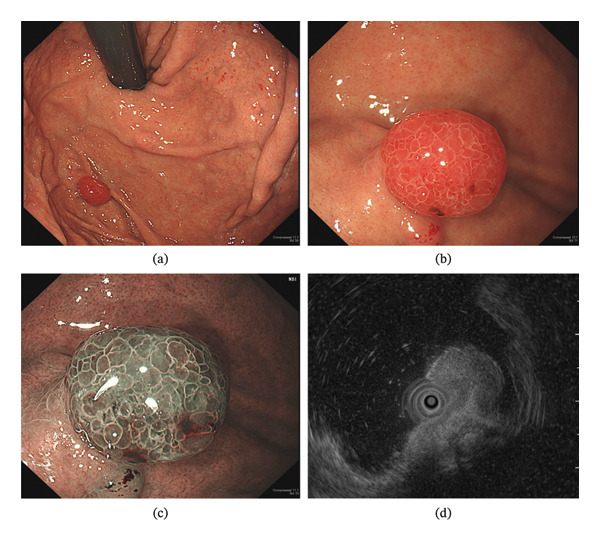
Endoscopic images from the 8‐month follow‐up examination. (a) The 15‐mm red polyp on the anterior wall had disappeared. (b) An 8‐mm raspberry‐like lesion was found where the Y‐III polyp had been previously located on the fundus. (c) Narrow band imaging. (d) Endoscopic ultrasonography.
